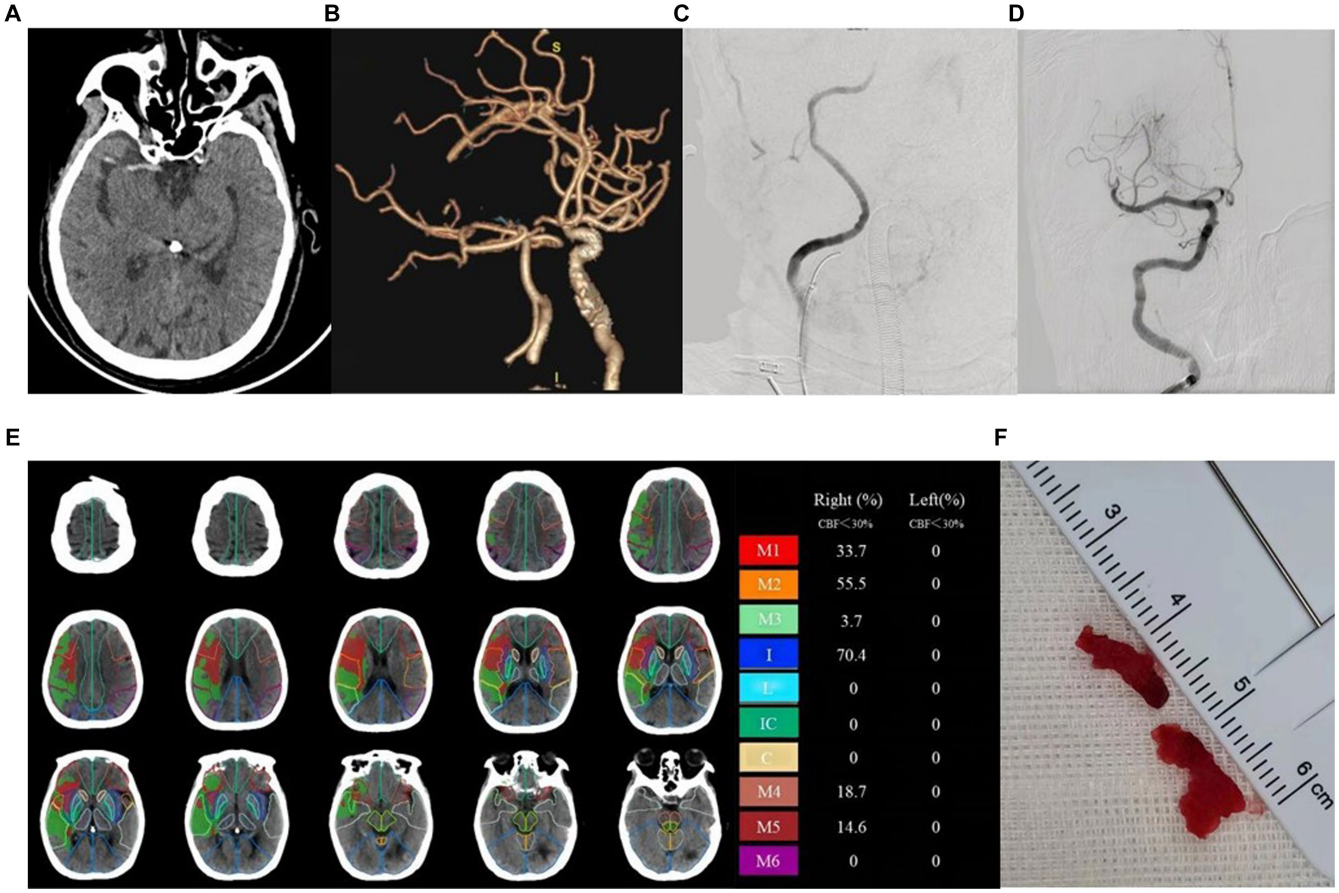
Example of patient with good prognosis (mRS≤2) and low ASPECT scores. Female, 78 years old, with limb weakness 1 day. ASPECTS is 4 points. (A) NCCT shows dense middle cerebral artery sign on the right side. (B) CTA MIP shows occlusion of the right internal carotid artery. (C) DSA shows stenosis of the right internal carotid artery. (D) DSA shows recanalization of the right internal carotid artery after surgery. (E) The core infarction involves the M1, M2, M3, internal capsule, M4, and M5 region, along with their respective infarction percentages. (F) Operation sample.
